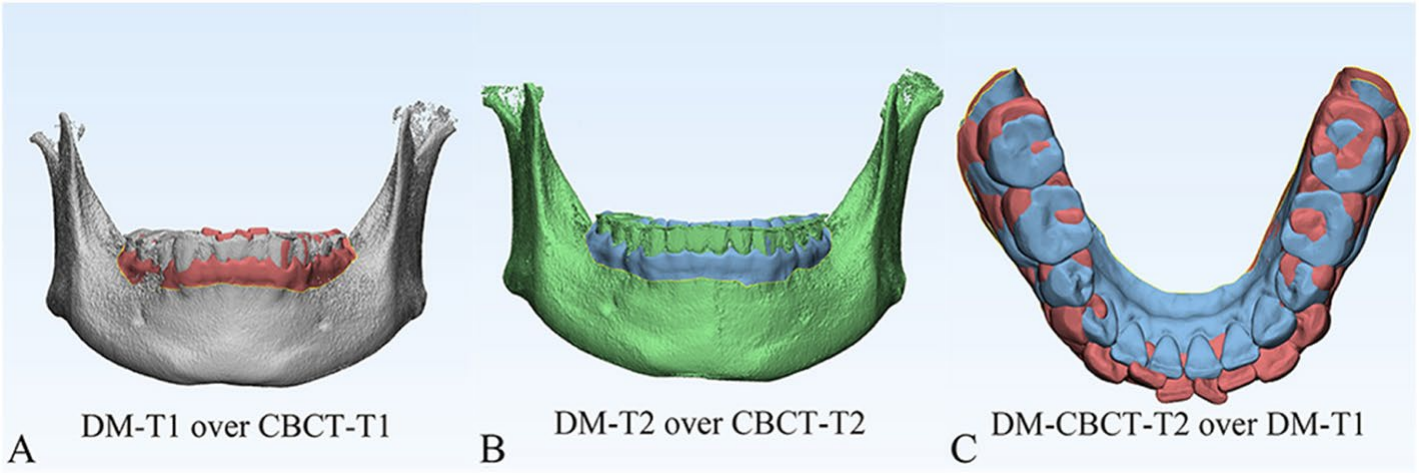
A Settle the position of DM-T1. B Registered the DM-T2 to CBCT-T2. C Acquisition of the CBCT-based DMs superimposition, DM-T1 (red) and DM-CBCT-T2 (blue)
